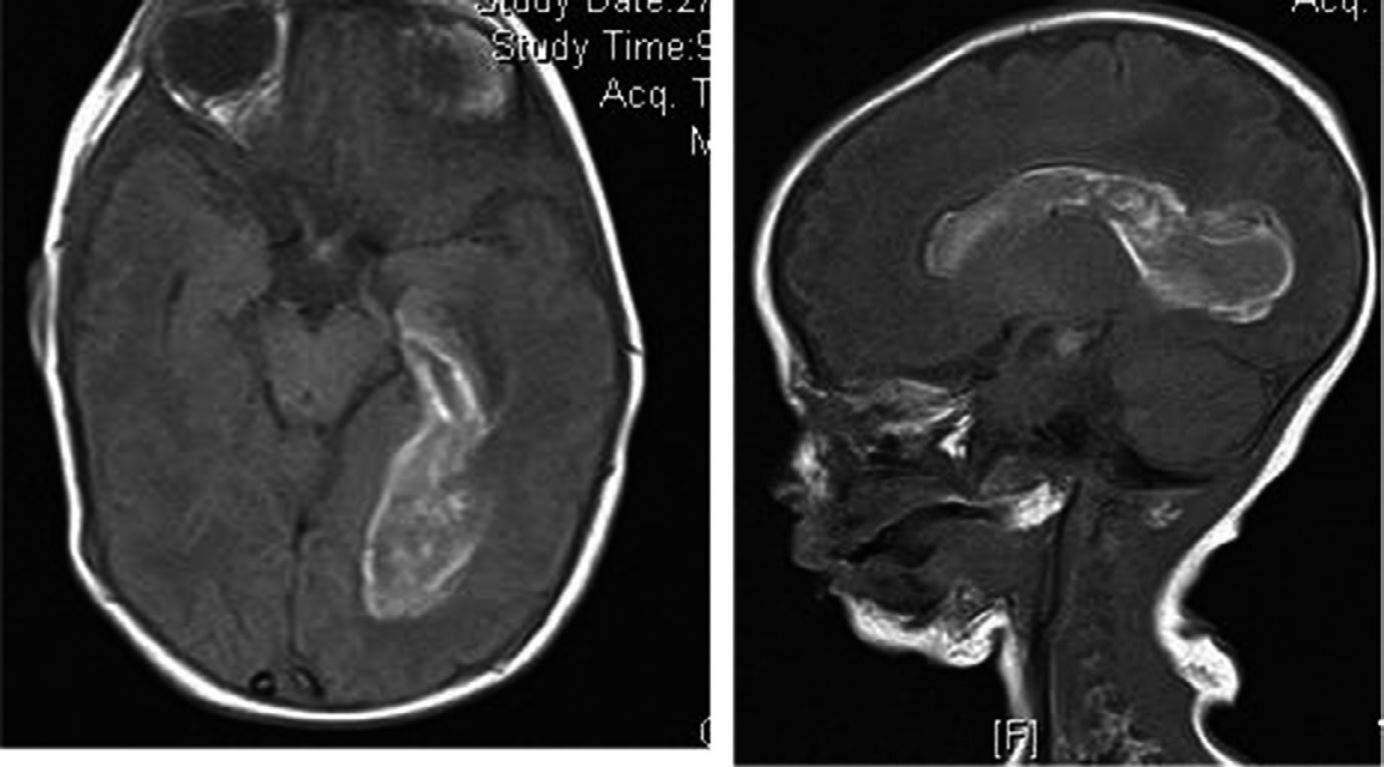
MRI of brain showing Intraventricular Hemorrhage
